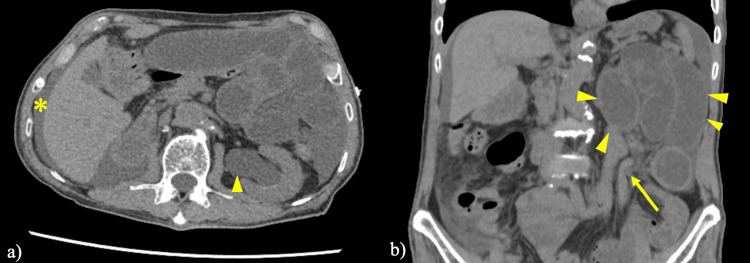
a: Abdominal axial plain CT. b: Abdominal coronal plain CT. a: Axial plain CT revealed ascites in the front of the liver (asterisk), and the hydronephrosis (arrowhead). b: Coronal plain CT showed dilated small bowel loops with the beak sign (arrowhead) and the left ureter (arrow).
